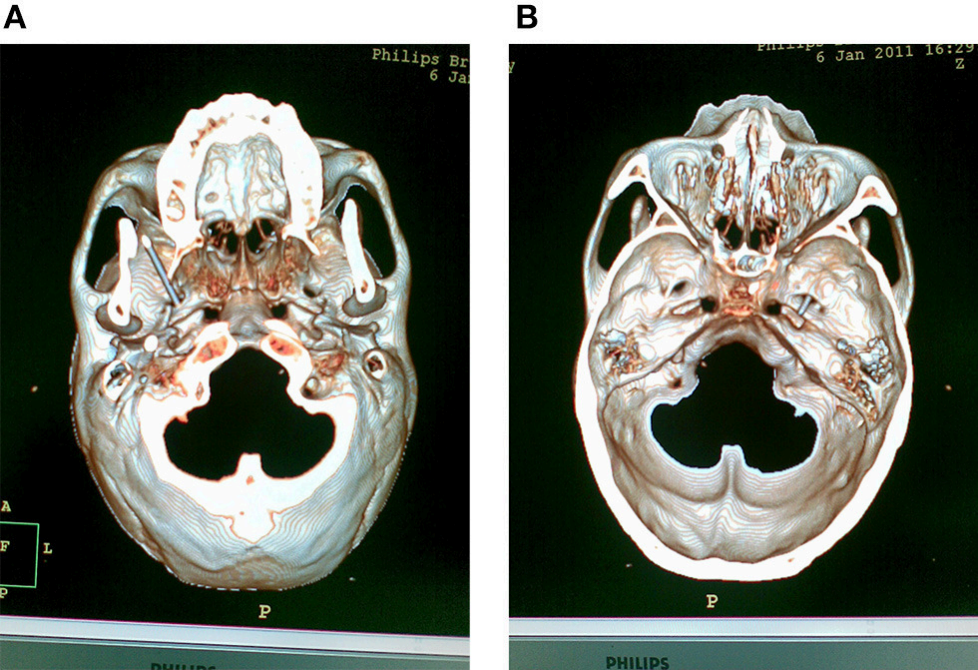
CT scans were performed to confirm the correct localization and the depth of the cannula tip into the foramen ovale. (A) The 3-D scaphion view image showed the localization of the cannula in the foramen ovale. (B) The 3-D image of the medial view of skull base showed the depth of the cannula tip into the foramen ovale.
